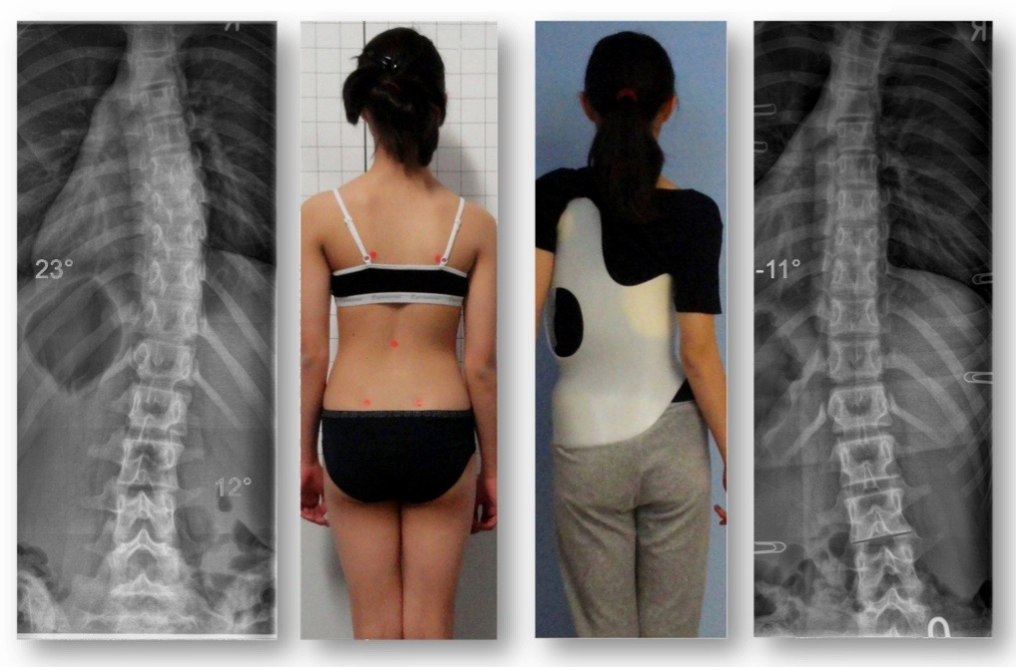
Intermediate result of the girl from Fig. (4) after 6 months of full-time treatment. At that time, she had outgrown her first brace and a second brace was made. As shown in the x-ray on the right, she achieved overcorrection in her second brace as well.
